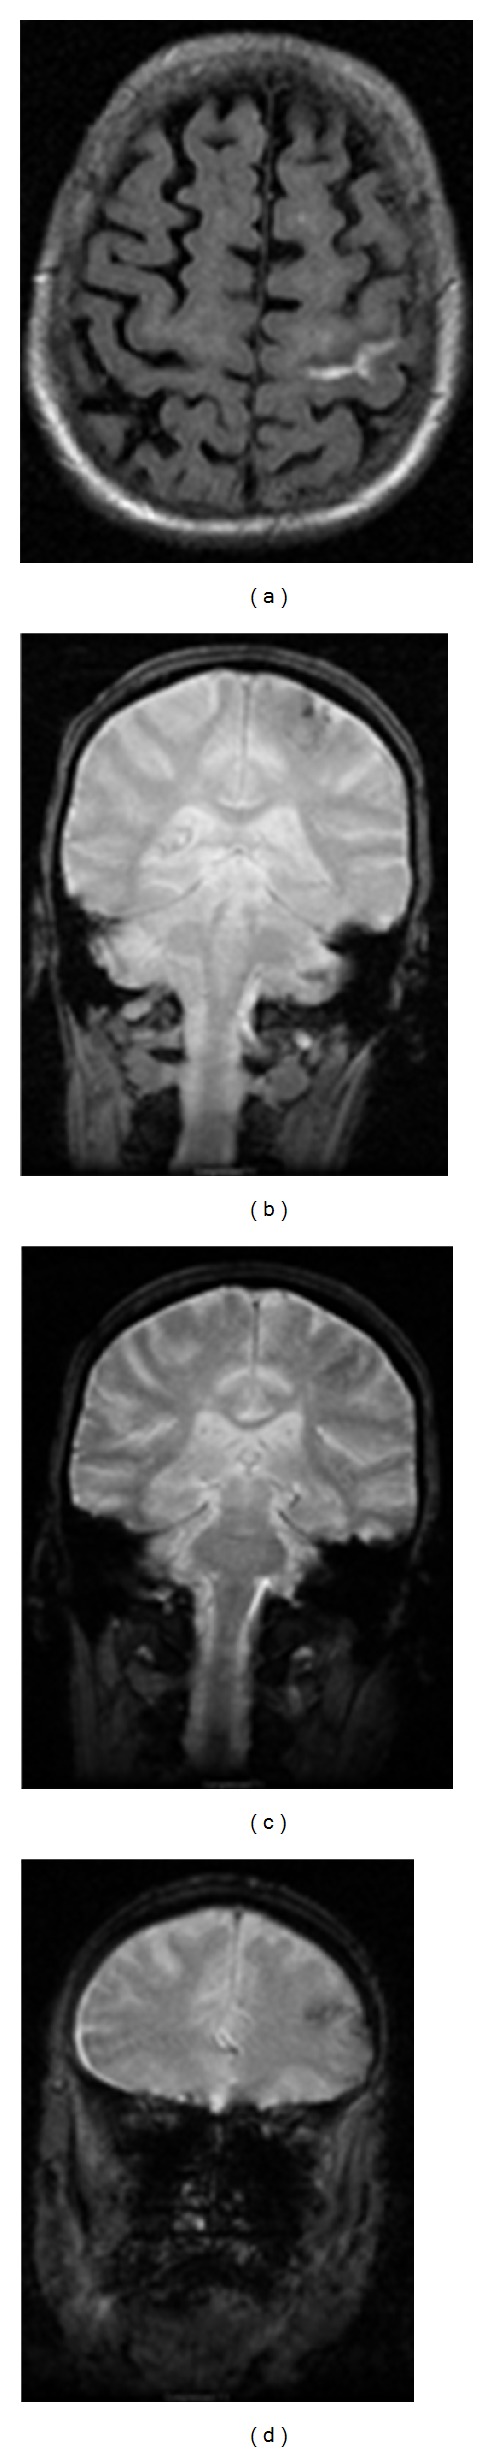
MR images of the brain. Axial FLAIR image (image a) of the index case confirms the finding of left central sulcus hemorrhage seen on the CT scan. Coronal gradient echo images (image b) demonstrate corresponding loss of signal within the left central sulcus. Coronal gradient echo images (images c, d) demonstrate signal loss within other adjacent cortical and subcortical areas consistent with hemorrhages and siderosis. Imaging findings and clinical presentation support the working diagnosis of cerebral amyloid angiopathy.
